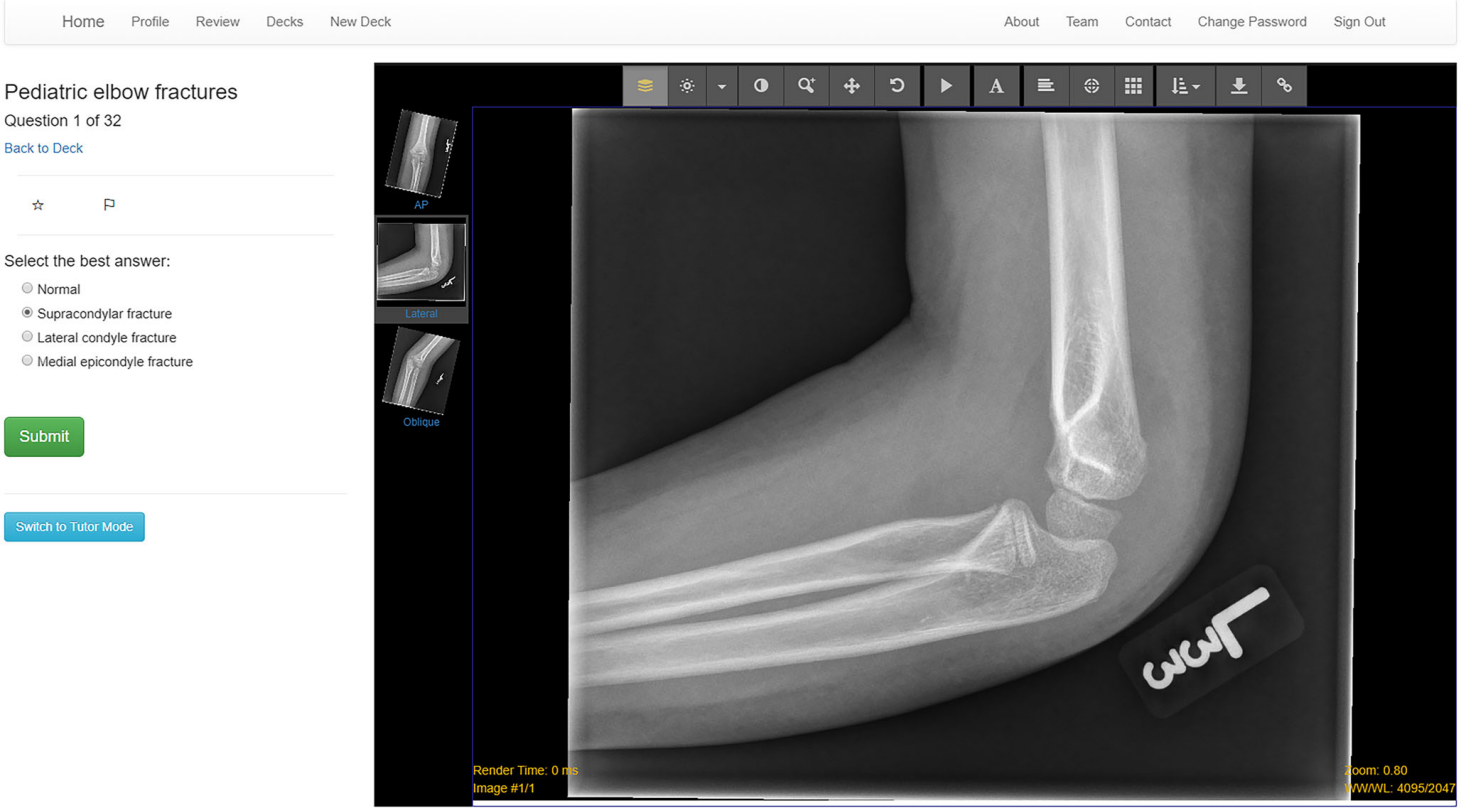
This screenshot demonstrates the user interface of one flashcard in a deck. This card demonstrates the limited number of diagnoses included in each deck (in this case four, including normal). Additionally, the integration with Pacsbin is demonstrated, in this case showing three images from one radiograph series
